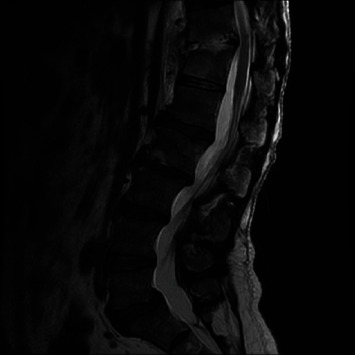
MR thoracic spine T2-weighted image demonstrates mild enhancement in the T11-T12 disc space with mild endplate erosion. No significant spinal stenosis.
